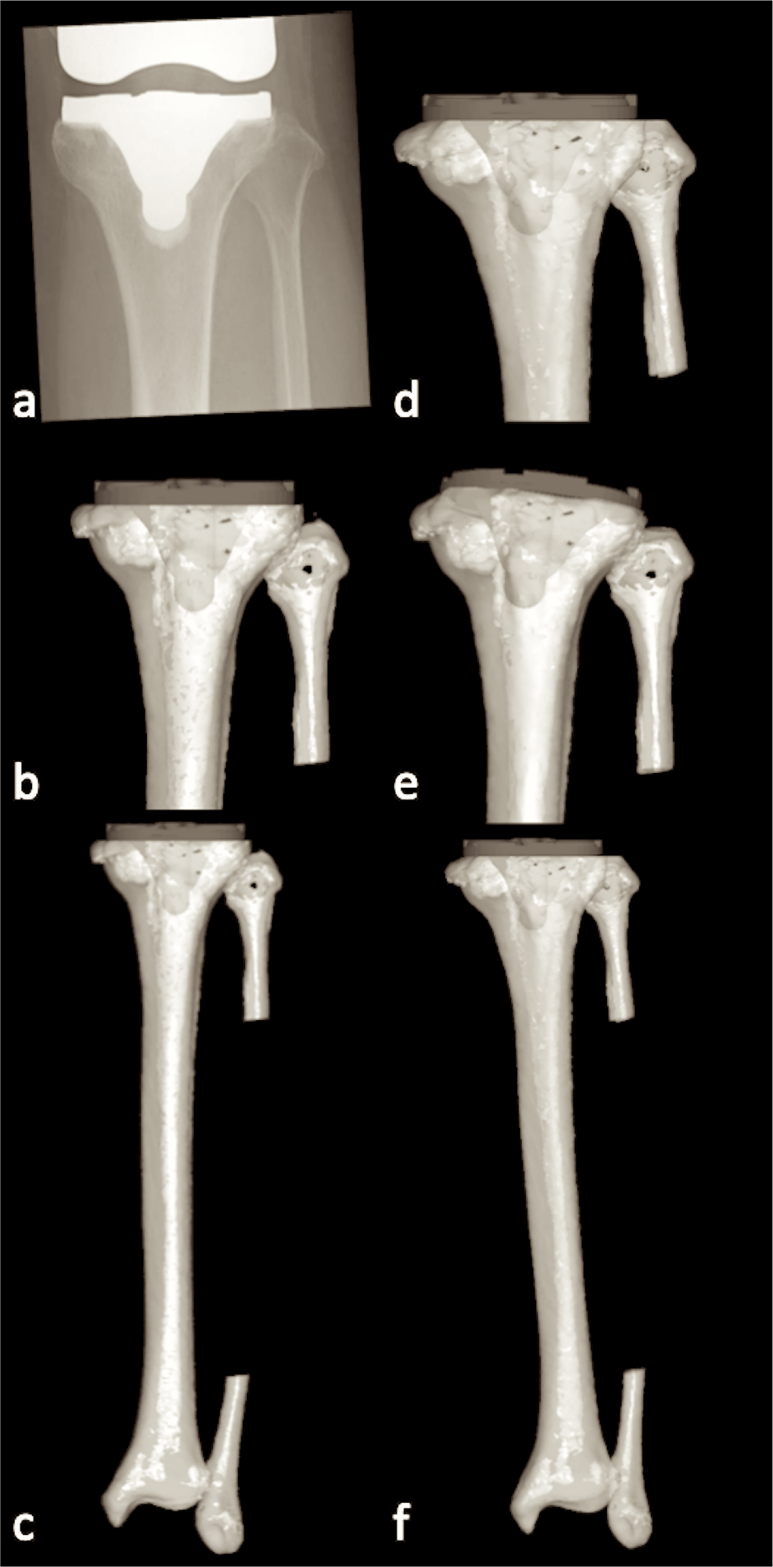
Pre-operative planning and post-operative evaluation. a Post-operative X-ray image. b Pre-operative planning of the tibial component. It can be seen that the rotational positional relationship between the tibia and fibula is different from the post-operative X-ray. c Pre-operative planning of the tibial component with the whole tibia. d Post-operative tibial component position. It can be seen that the rotational positional relationship between tibia and fibula is the same as the post-operative X-ray (a), but different from the pre-operative planning X-ray (b). e Post-operative tibial component positioning presented by the co-ordinates’ system as pre-operative planning. It can be seen that the tibial component is placed in more valgus, posterior slope and internal rotated position than the pre-operative planning (b). f Post-operative tibial component position with the whole tibia
